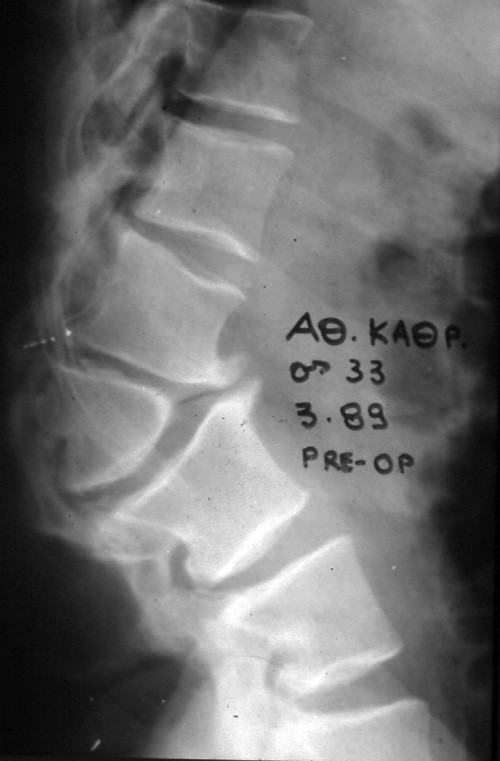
Plain lateral radiograph of patient's lumbar spine showing kyphosis of 70 at the level of the 2^nd ^lumbar vertebral body.
